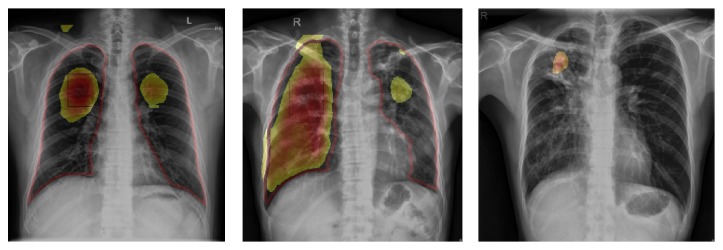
Saliency maps for X-rays from Shenzhen No. 3 People's Hospital.
